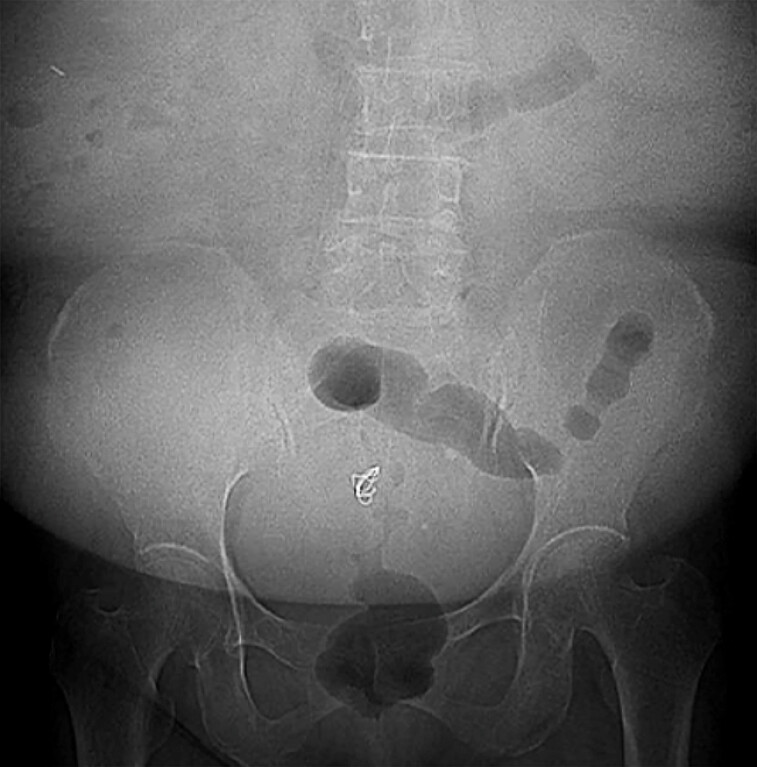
Post-procedural plain radiograph showing the coil in the pelvis.
